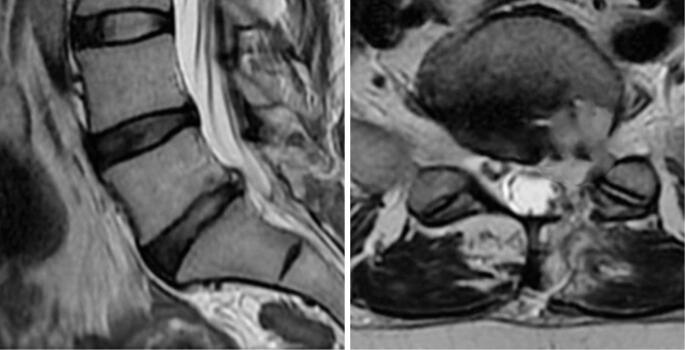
Postoperative MRI demonstrating microdecompression with left hemilaminotomy of L5 and flavectomy, along with edema in adjacent myoadipose planes and S1 root thickening. The previously identified extruded disc herniation compressing the nerve root is no longer present
